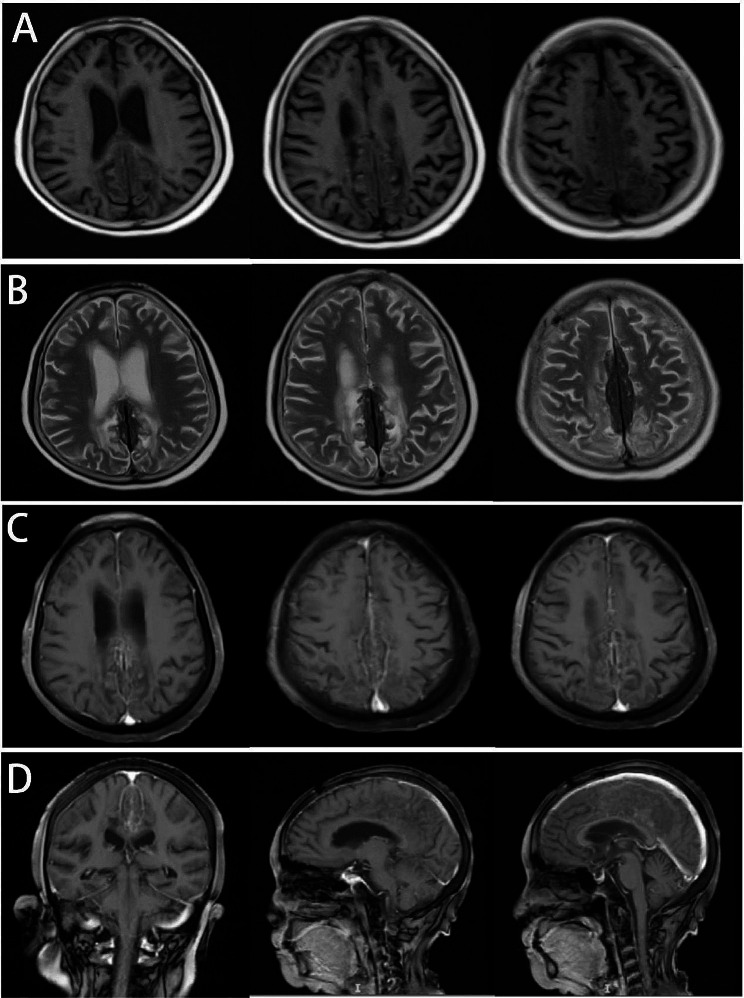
Cerebral brain MRI of the index patient one year after treatment. Both T1WI (A) and T2WI (B) show that the irregular fusiform thickening of the falx cerebri is smaller than before, and the patchy high-signal edema area around the lesion is significantly reduced (B). Compared with the enhanced scan before treatment, the enhancement was not apparent (C, D)
